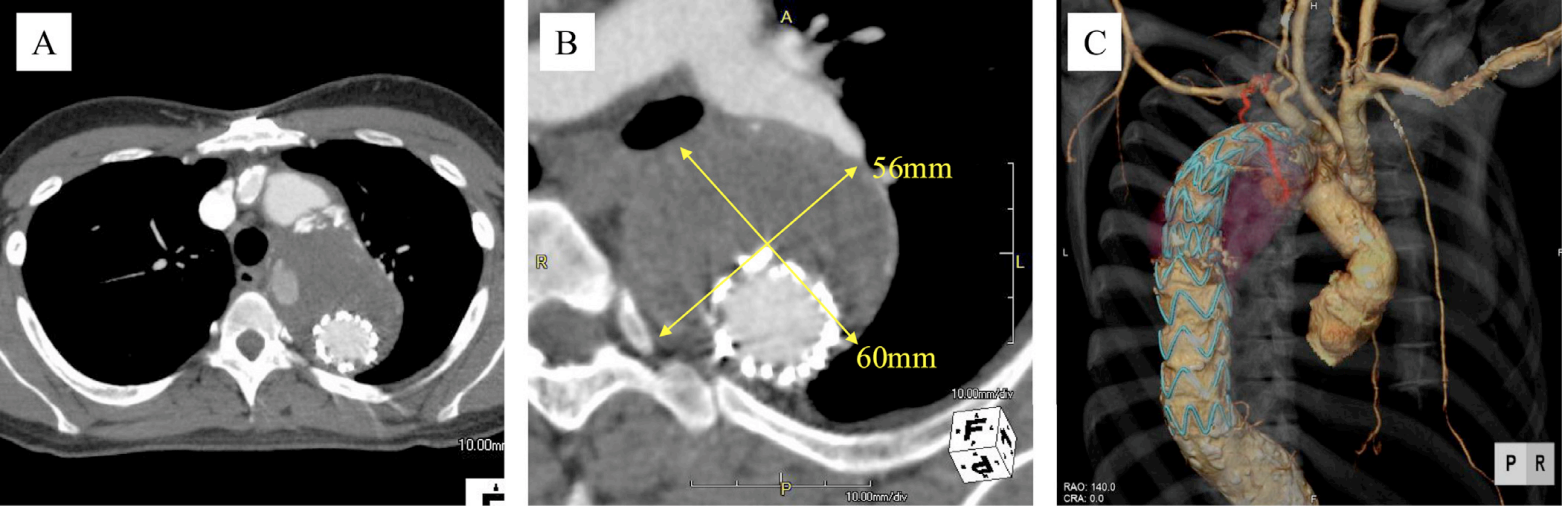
Computed tomography (CT) in Case 3. (A) An endoleak was identified in the proximal ascending aorta. (B) Aneurysm enlarged to approximately 60 mm in diameter (2 mm enlargement over 6 months). (C) Feeder vessel coursed along the left vertebral body and formed connections with the bronchial artery, costocervical artery, and left subclavian artery.
